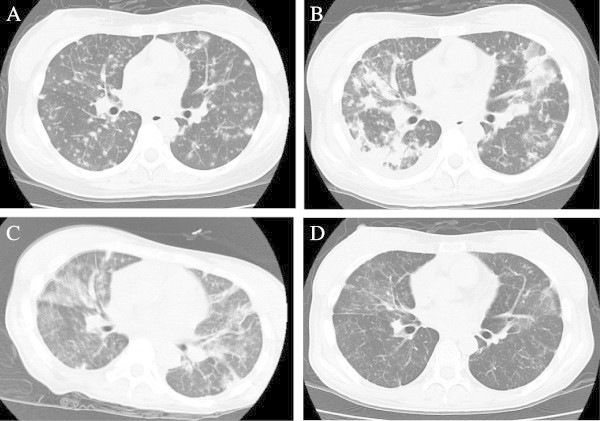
Chest computed tomography scan. A. Scan on admission. B. Scan during chemotherapy (on day-28 before allogeneic transplantation). C. Scan on day +61 after allogeneic transplantation. D. Scan on day +195 after allogeneic transplantation.
